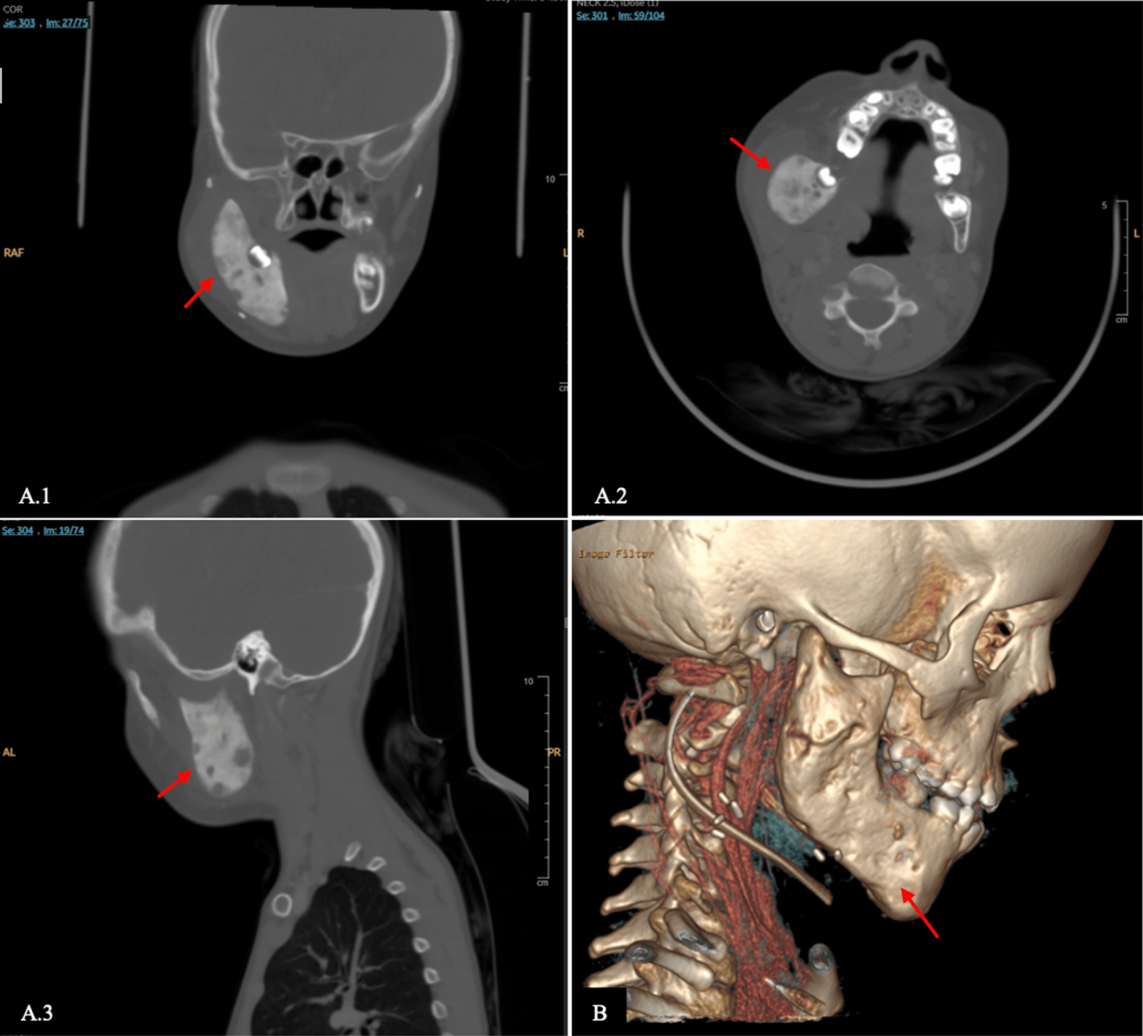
Computed tomography (CT) scan showing a lesion centered within the right mandibular ramus, extending to involve the coronoid and condylar processes, and anteriorly to the mandibular body near the symphysis menti. A.1: Pre-operative CT scan (coronal view); A.2: axial view; A.3: sagittal view. B: Three-dimensional CT. The red arrow shows the lesion.
